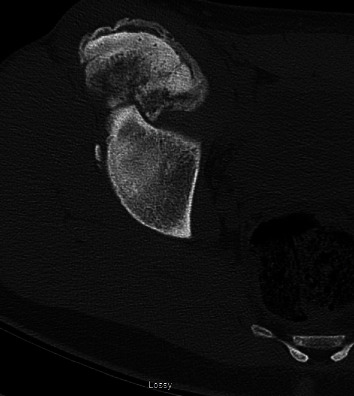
Axial CT scan demonstrating the large amount of bone deposit from the right AIIS avulsion fracture.
